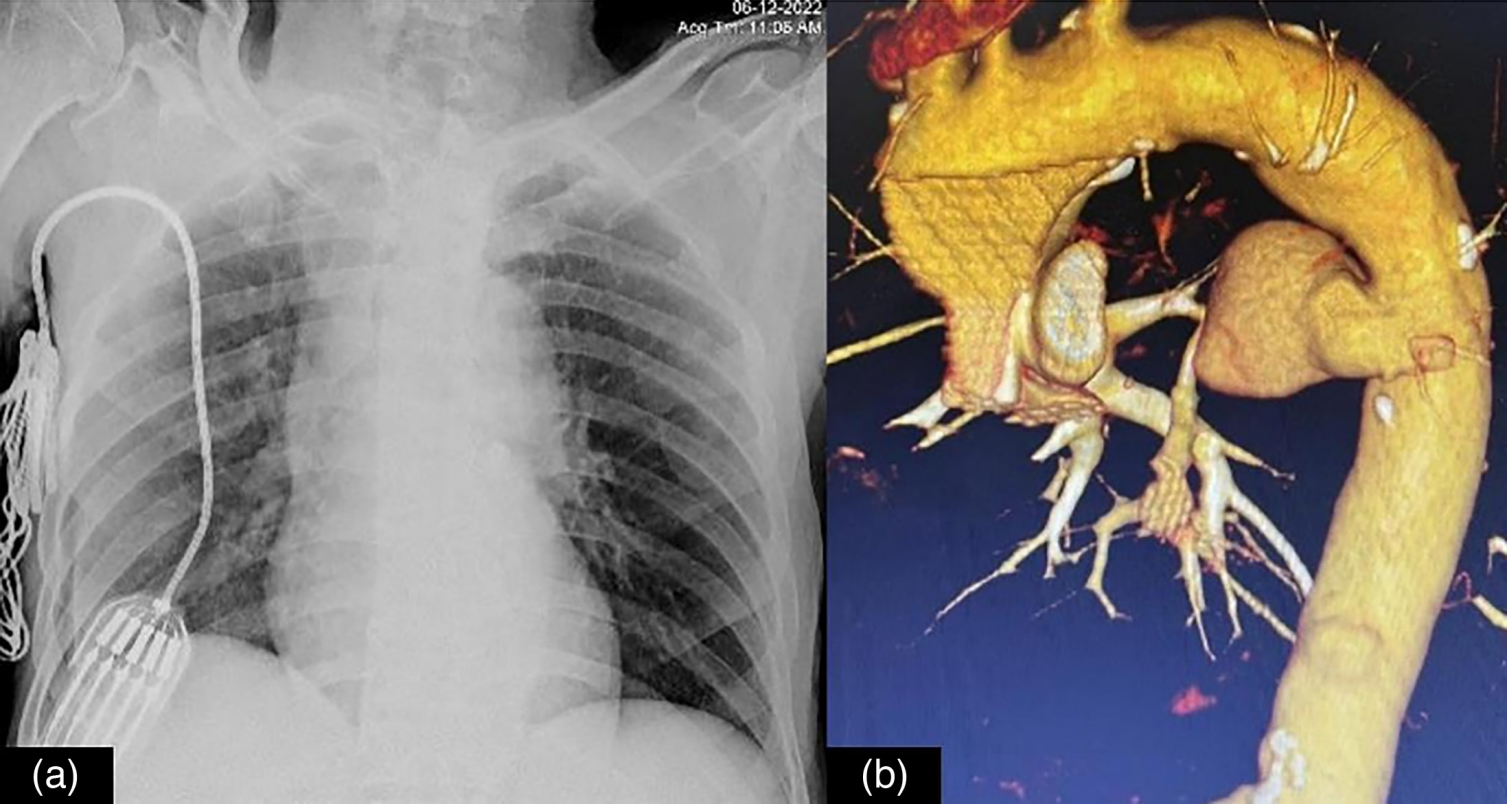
Chest radiograph in a a 70-year-old gentleman showing mediastinal widening (a). His aortogram subsequently revealed a rupture descending thoracic aortic pseudoaneurysm (b). His blood cultures were positive for *Pseudomonas aeruginosa*.
